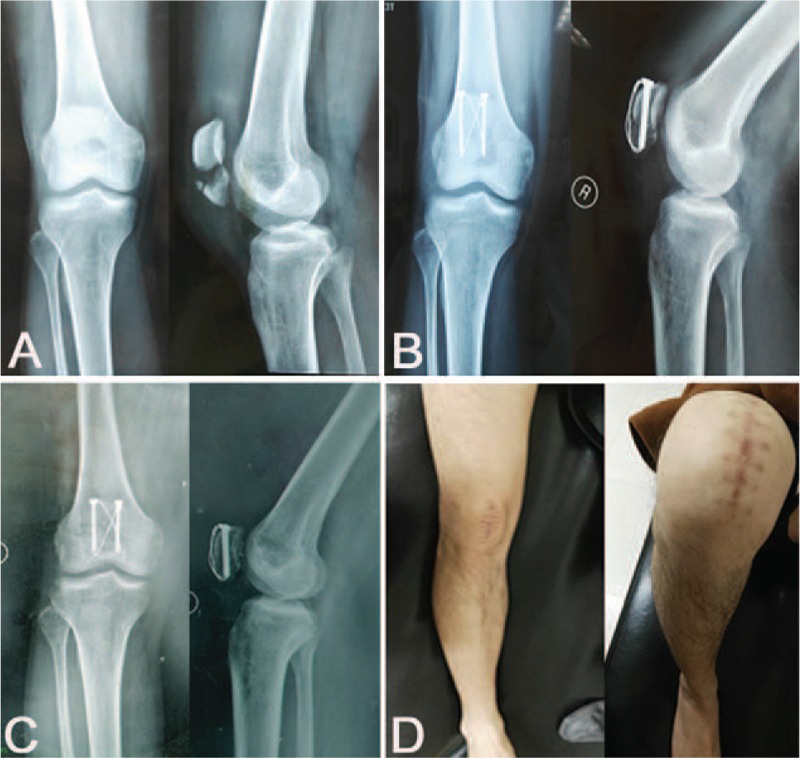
A 48-year-old male patient with right transverse patella fracture underwent CSTB fixation. X-ray film showing transverse patella fracture (A). Postoperative film showing anatomical reduction of the fragments and stable fixation (B). No loosening and displacement of the cannulated screw and steel wire 16 months after operation (C). Excellent knee functions (D). CSTB = cannulated screw tension band.
